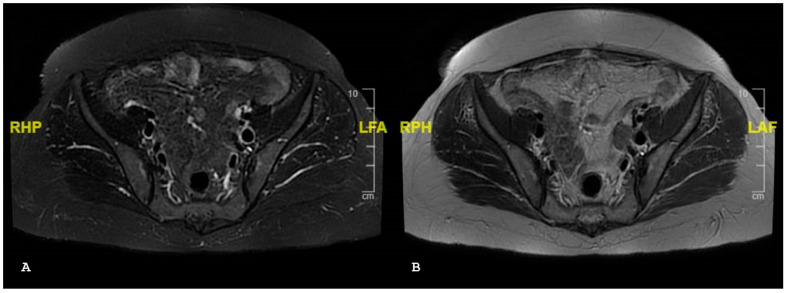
Axial short tau inversion recovery (A) and axial T1-weighted magnetic (B) resonance imaging sequences show moderate subchondral sclerosis (a map morphology), surrounded by a thin rhyme of bone edema.
